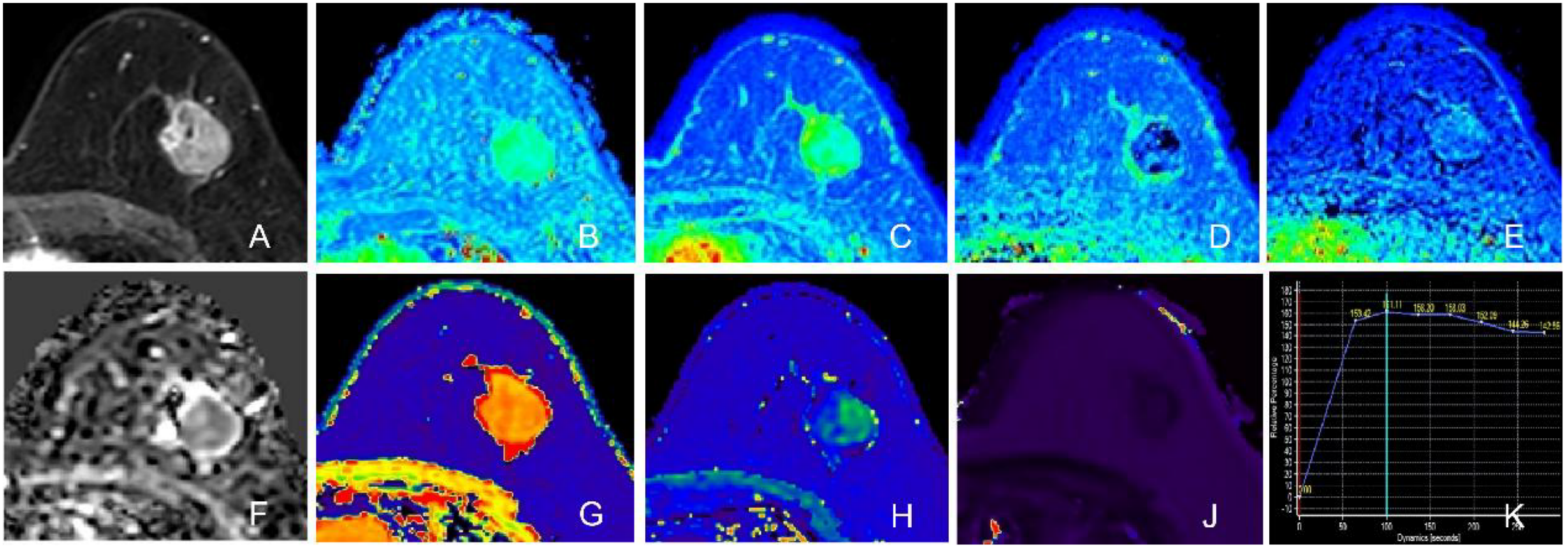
Representative images of a Triple-Negative Breast Cancer (TNBC) lesion (Invasive Ductal Carcinoma, Ki-67: 60%) in the left breast. The tumor exhibits a round shape with smooth margins and heterogeneous enhancement. (A) Axial DCE-MRI image. (B) Relative Enhancement (RE) map. (C) Maximum Enhancement (ME) map. (D) Wash-in rate (WI) map. (E) Wash-out rate (WO) map. (F) ADC map showing restricted diffusion. (G) Native T1 map. (H) Enhanced T1 map. (J) T2 map. (K) Time-Intensity Curve (TIC) showing an outflow pattern (Type III).
